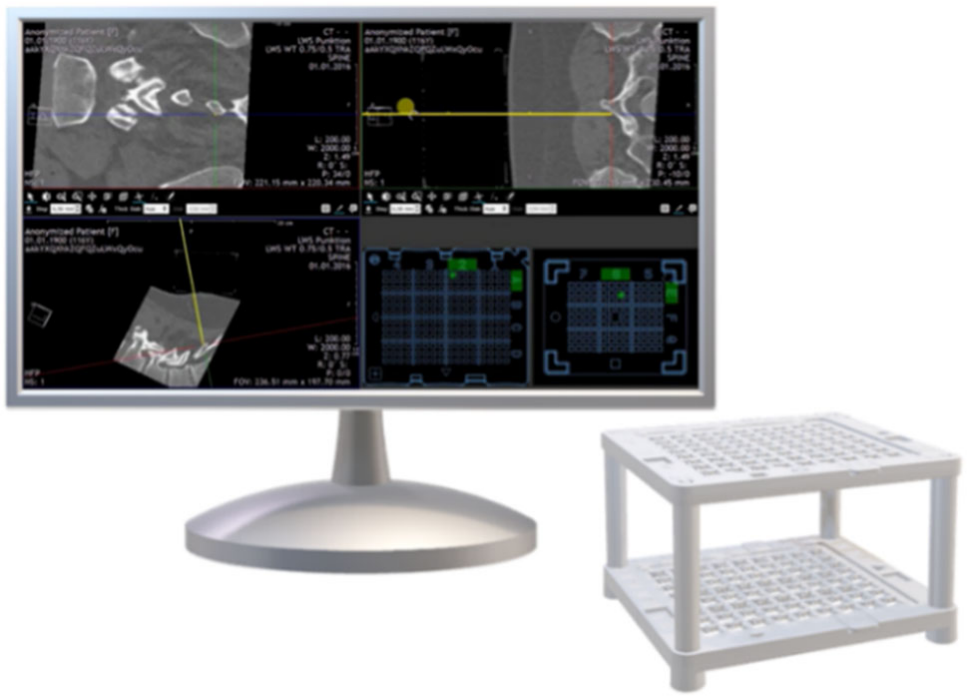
Medical Templates planning software and Navigation Cube. The yellow line visible in CT-scans represents a virtual needle which is used to plan the puncture. As the needle moves, a green dot representing the coordinates in the top and bottom plates (shown in the bottom right quadrant) automatically updates. The required angle is achieved by inserting the needle through the corresponding holes and corners on the top and bottom plates of the Navigation Cube
